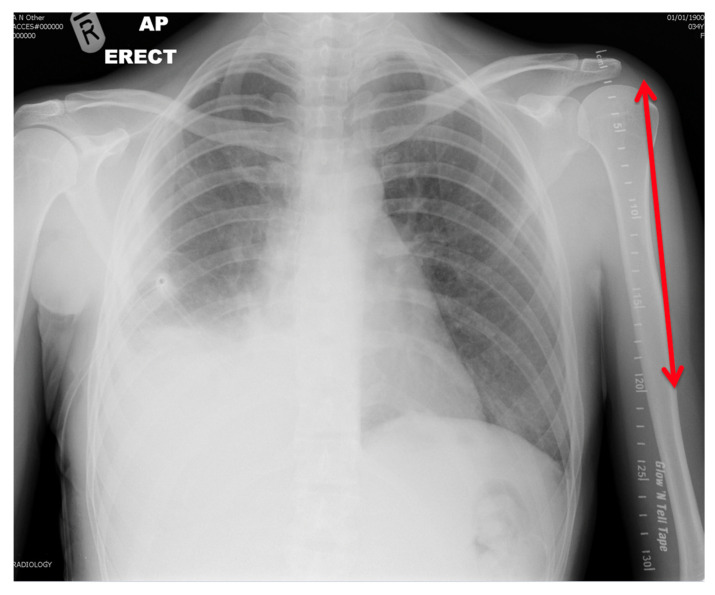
Radiograph demonstrating radio-opaque marker parallel to left humerus.
